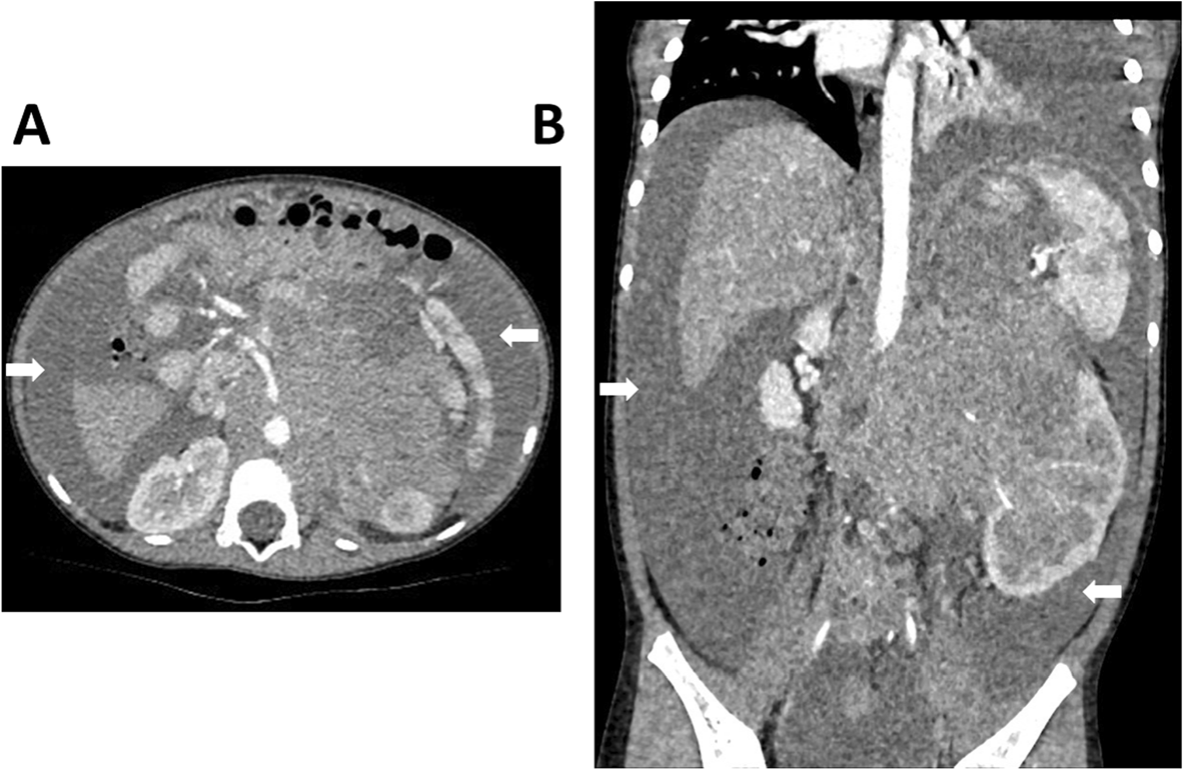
Abdominal enhanced computed tomography (CT) imaging findings of ruptured neuroblastoma. a Transverse section image. b Coronal reformatted image. The neuroblastoma in the left adrenal region is irregularly shaped, with no clear margins and hypodensity in the surrounding area, which is highly suggestive of tumor rupture (arrows denote the hypodense region)
